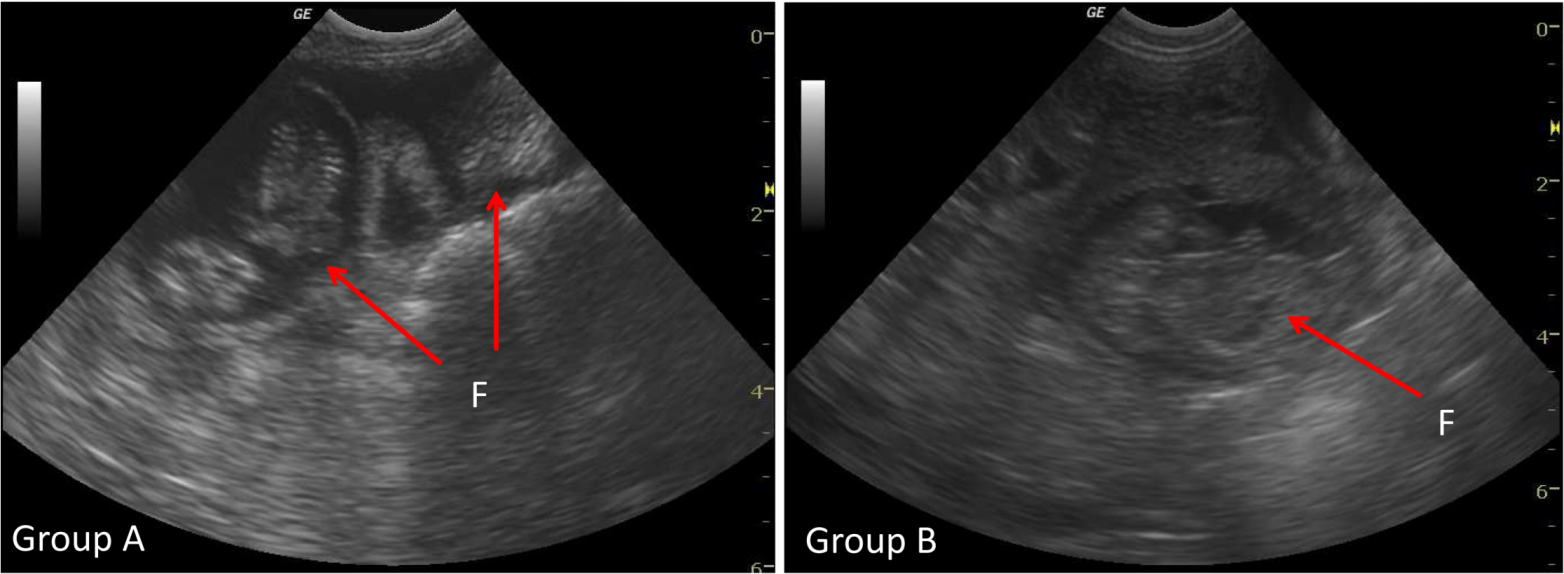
Ultrasound pregnancy diagnosis. At Day 21 post mating, by counting the number of foetuses (F). Test (Group A) and control group (Group B).
